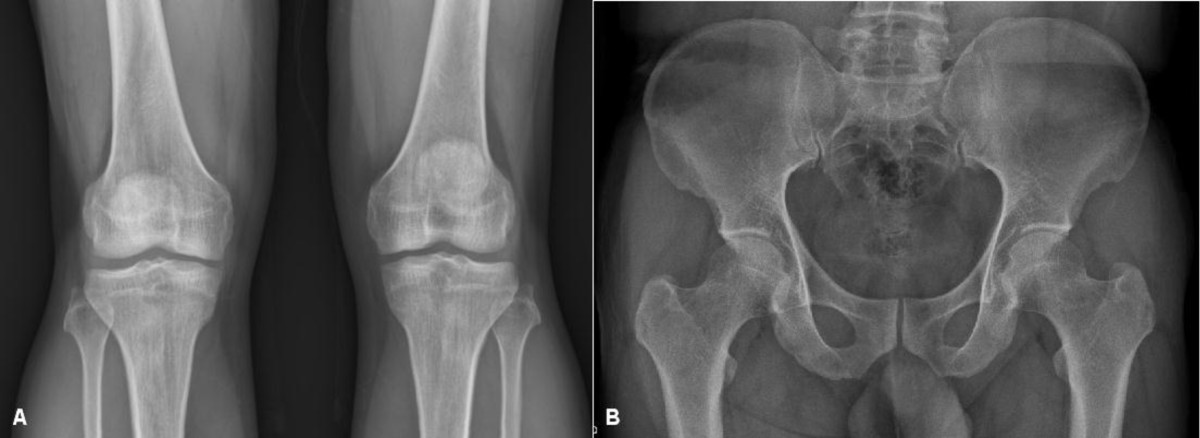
Radiographs of the proband’s father at the age of 41 years. Anteroposterior view of knee joints revealed early osteoarthritis with findings including of medial joint space narrowing and joint surface irregularity (A). The capital femoral epiphysis of the proband’s father has no evidence of dysplasia (B).
